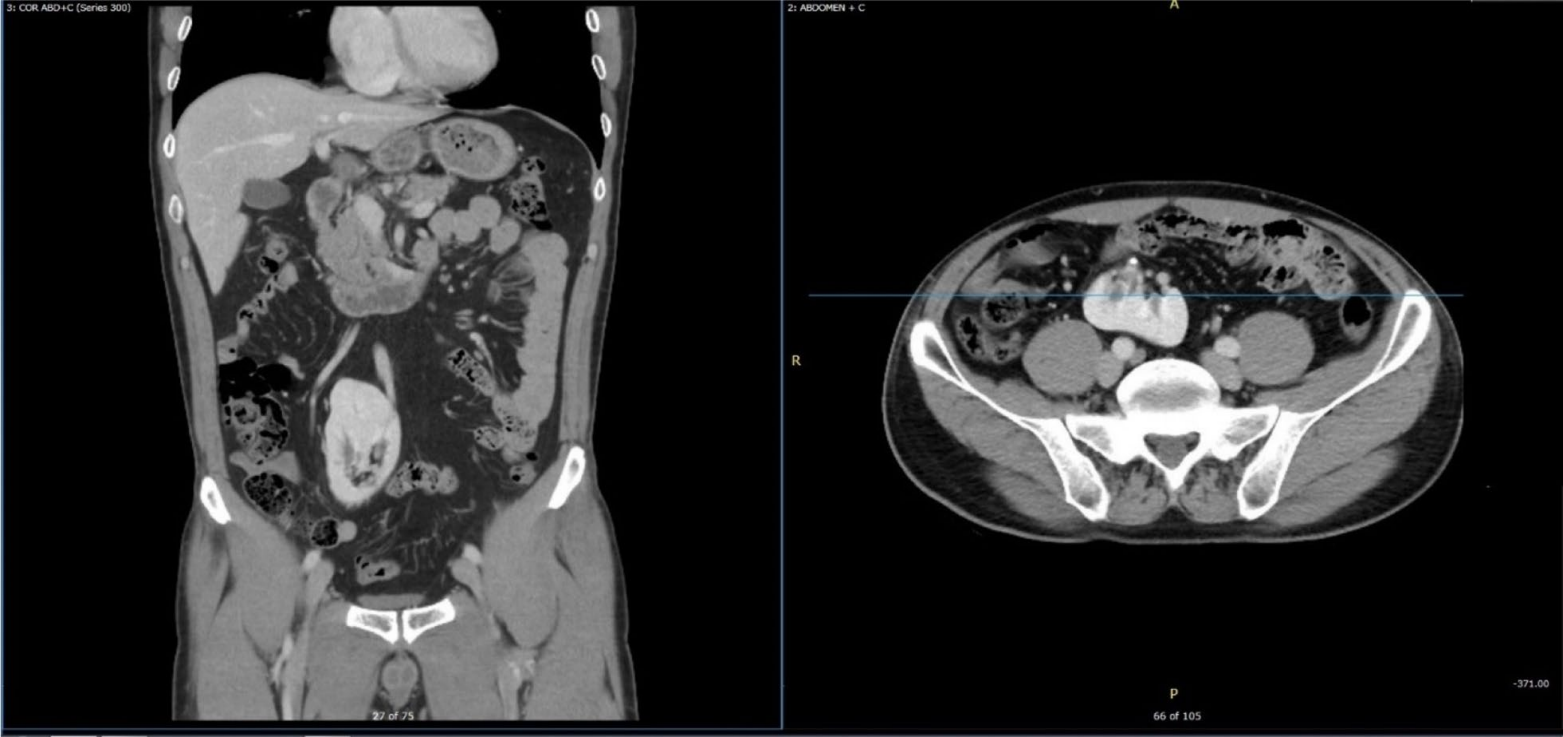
CT abdomen, showing anterior malrotated ectopic right kidney with small stone at pelviureteric junction
